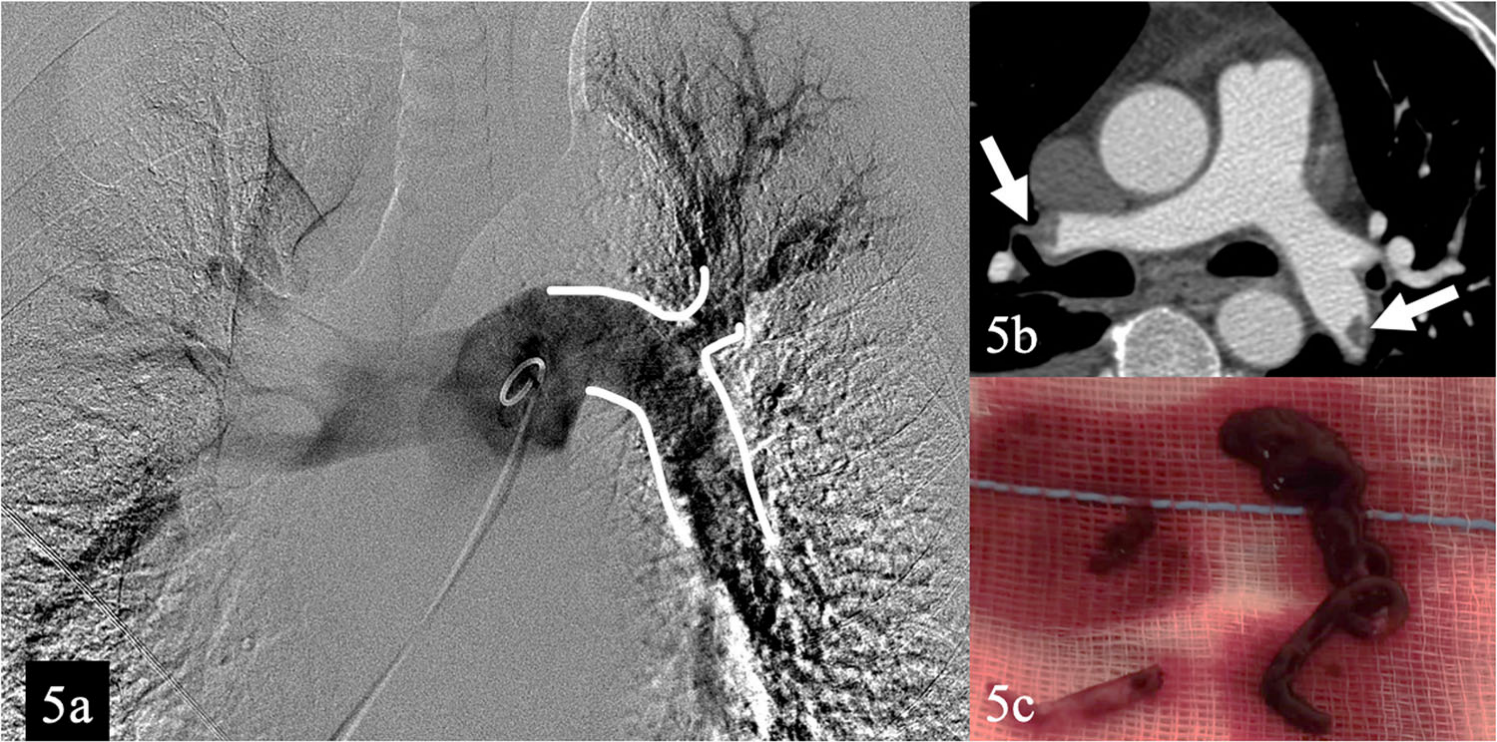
Previous patient (a) Pigtail angiogram confirms satisfactory clot extraction with patency of the pulmonary arteries (white draw line). Significant reduction of pulmonary artery pressure and RV/LV was observed at 48 h. b Computed tomography angiogram confirms only small volume residual thrombus (arrows). c Chronic and acute clot elements were extracted
